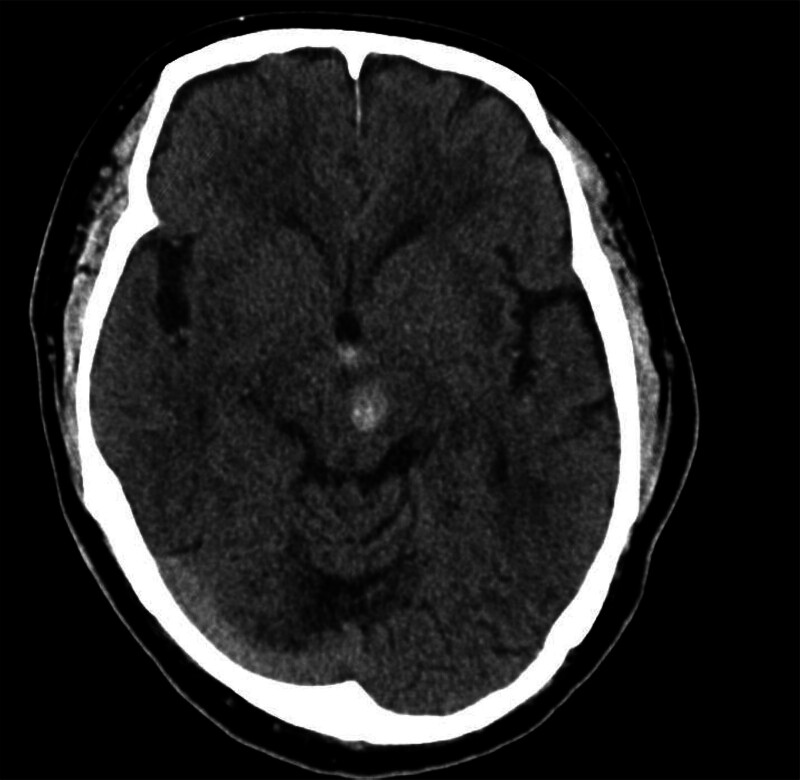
CT image of midbrain hemorrhage.
